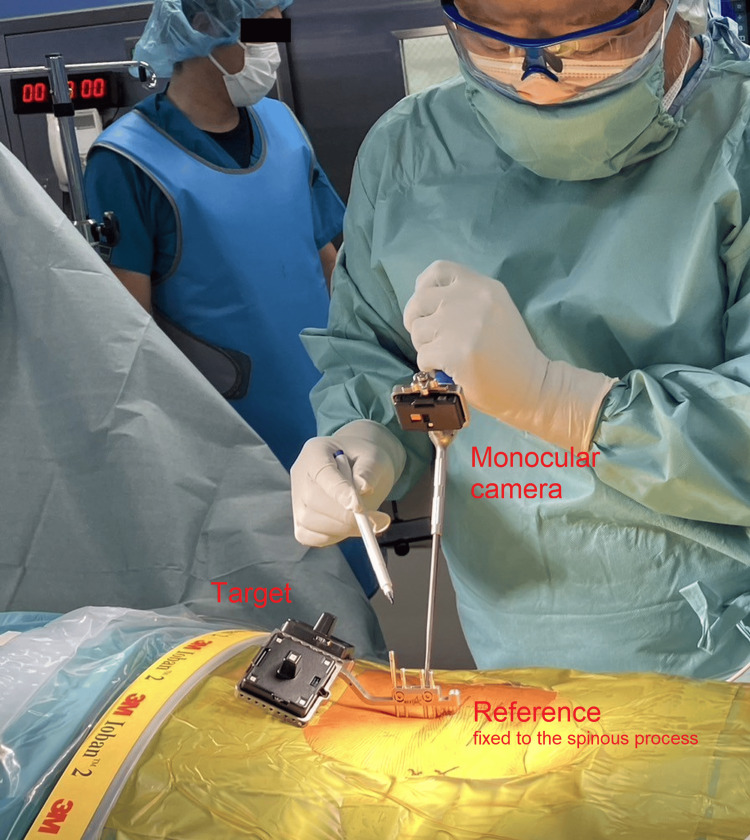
Intra-operative photographs of the navigation system in use The target is mounted on the reference fixed to the spinous process, and the monocular camera attached to the probe recognizes the target, transmitting positional information via Bluetooth to the control unit. Dr. Takeshi Umebayashi is holding the probe with the attached monocular camera. The surgeon can perform the procedure while confirming the real-time position of the probe on the 3D field displayed on the control unit, without the need for fluoroscopy. Conventional navigation systems rely on tracking balls attached to both the reference and the probe, which are recognized by an external camera outside the surgical field to determine positional information. In contrast, this system completes positional tracking through direct interaction between the reference and the probe. At this stage, intraoperative CT imaging has already been completed, and the fiducial has been removed.
